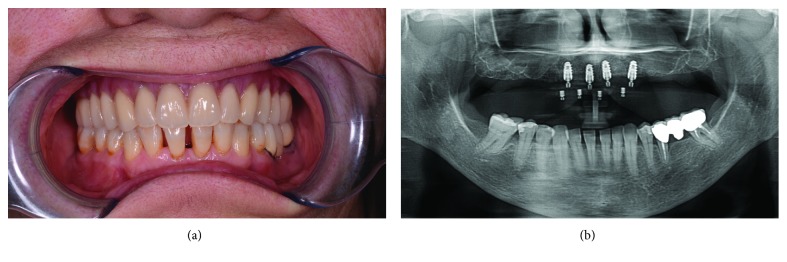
Clinical and radiographic control at 1 year from implant placement. (a) Frontal clinical photo; (b) panoramic radiograph.
